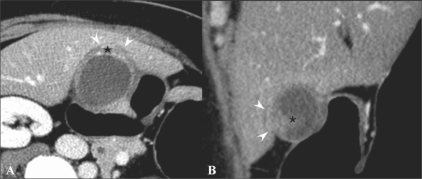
A 54-year-old woman with gallbladder cancer. Axial CT scan (A) shows eccentric wall thickening and a papillary lesion (*) in the fundus of the gallbladder. The fat plane between the liver and the gallbladder seems to be preserved in the axial plane (arrowheads). This lesion was interpretated as T2 on the axial CT image. Oblique coronal MPR image (B) reveals focal hepatic invasion (arrowheads), thus changing the staging and management. Asterisk indicates the papillary lesion
